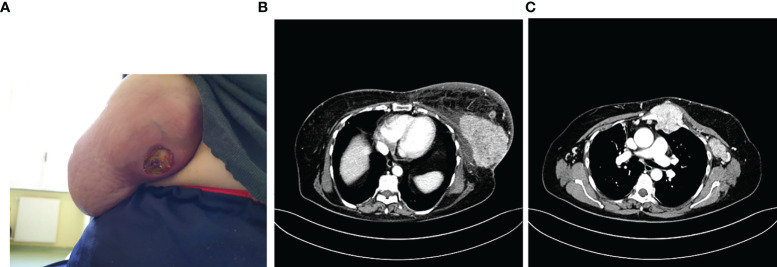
Photo of the ulcerated swelling at the level of the external quadrants of the left breast (A), described also at the CT scan (B) with the involvement of locoregional lymph nodes and sternum (C).
